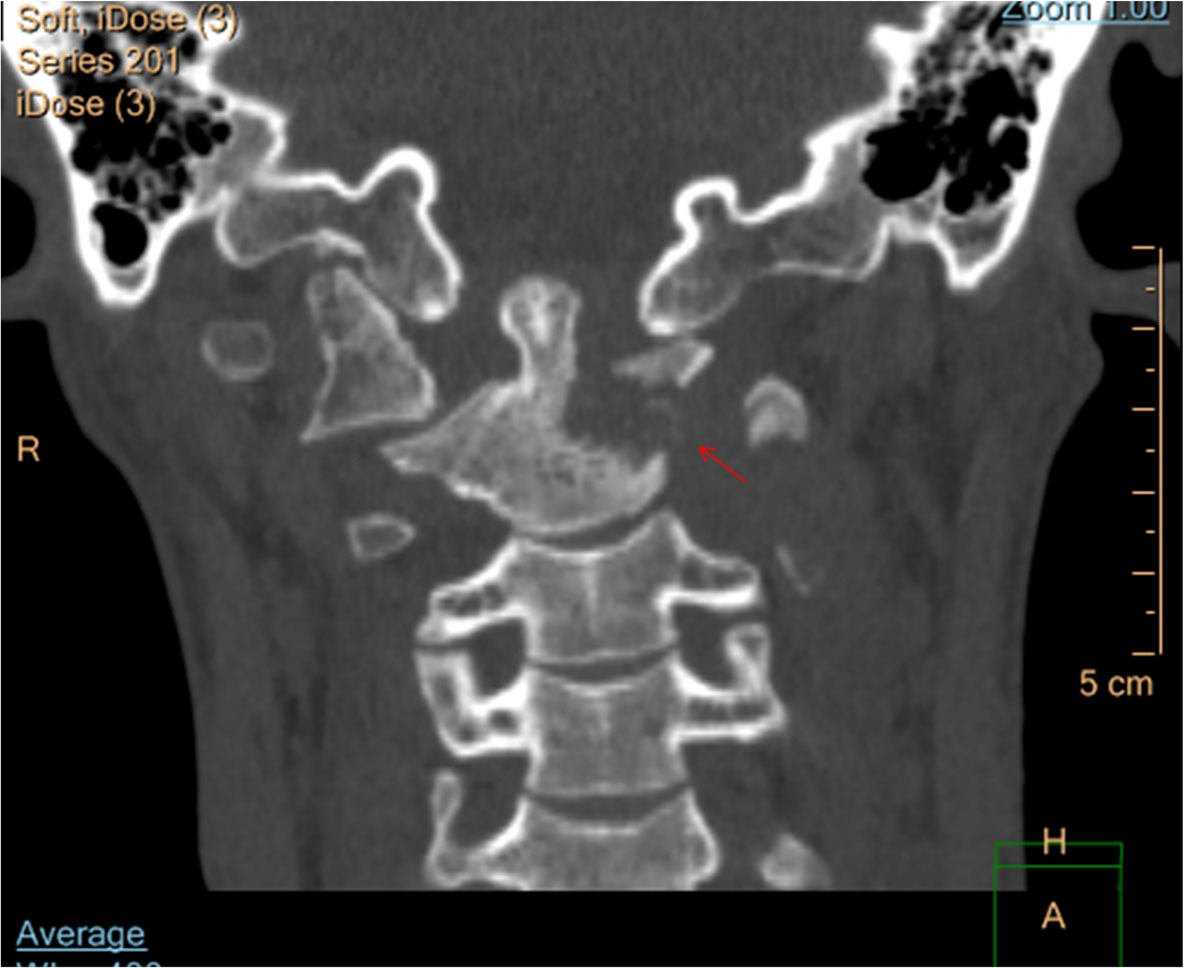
Preoperative CT scanning showed severe damages at the odontoid process and the vertebral body on the left side
